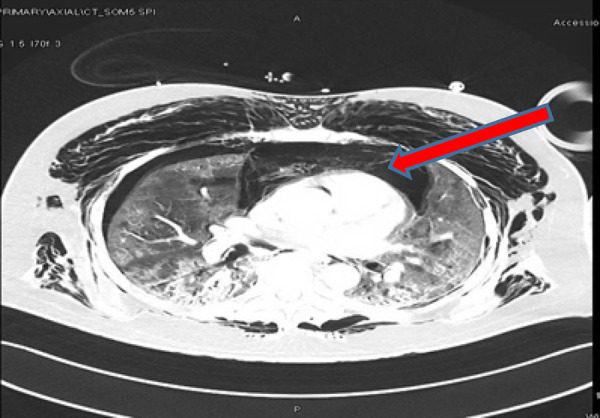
Computed tomography of the chest showing tension pneumomediastinum with pneumothorax
